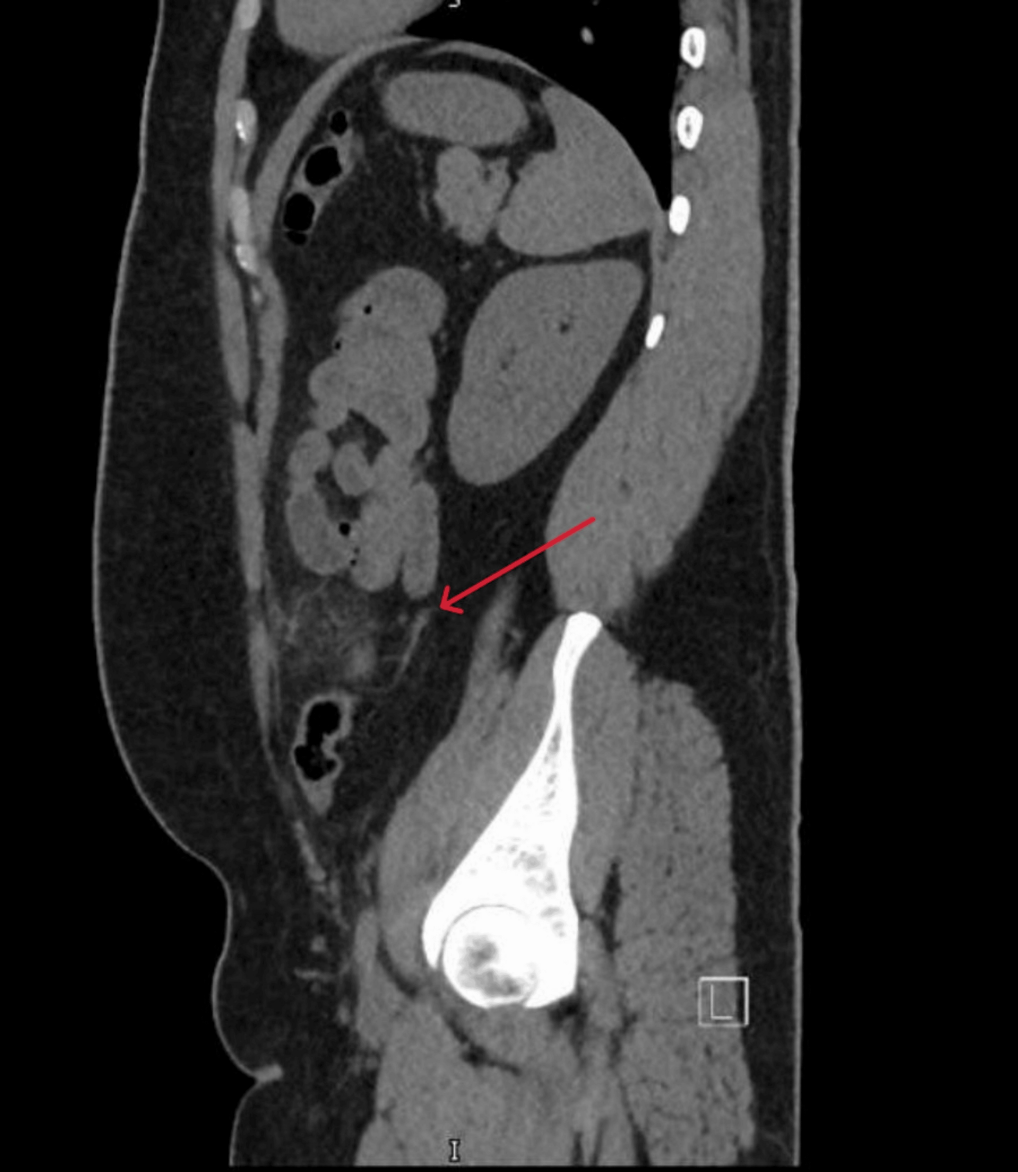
Sagittal computed tomography image of descending colon epiploic appendagitis The sagittal computed tomography image demonstrates an ovoid fat-density lesion adjacent to the lower descending colon, surrounded by inflammatory fat stranding (red arrow), consistent with epiploic appendagitis.
